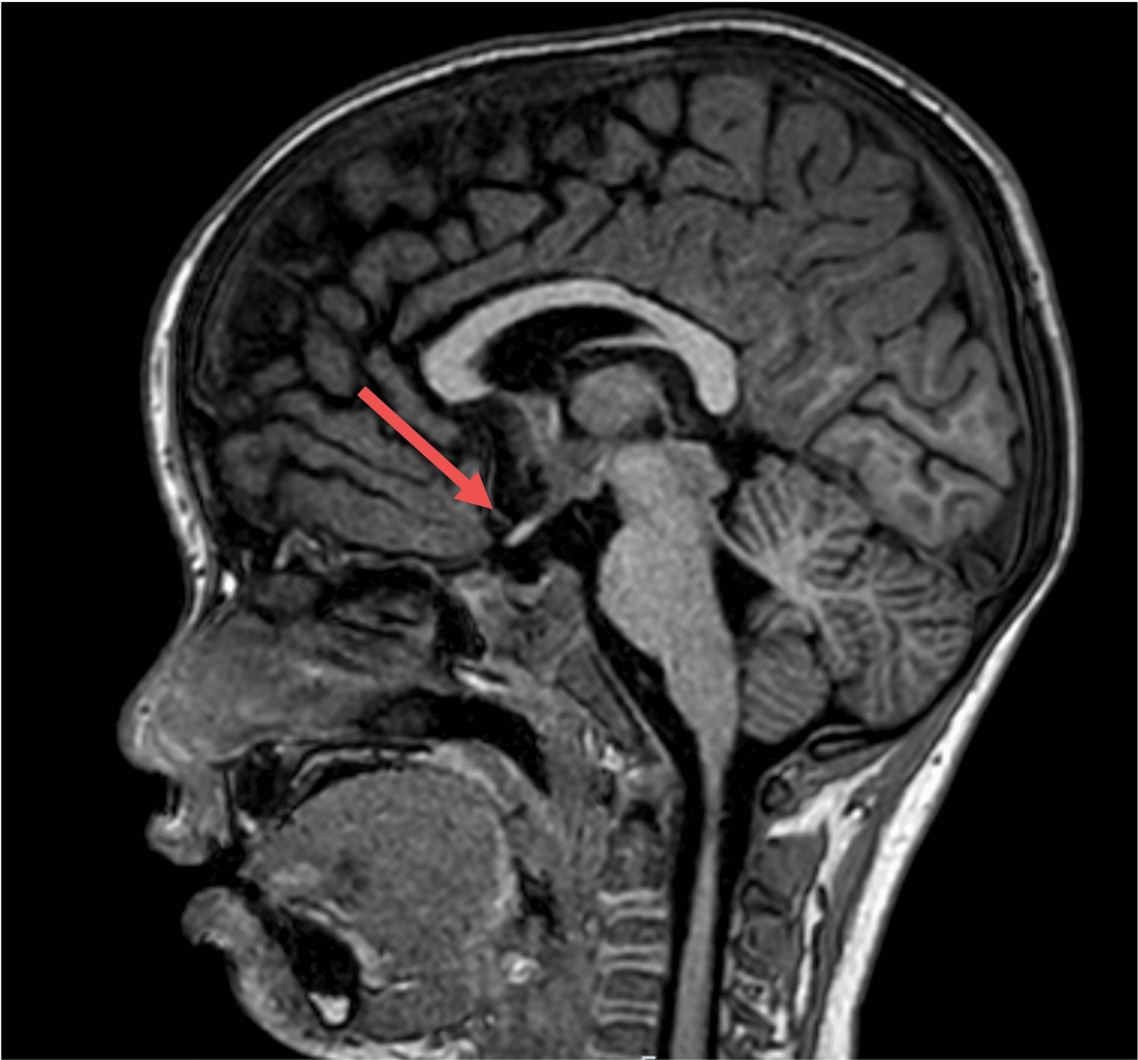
Post-therapeutic magnetic resonance imaging of the brain Magnetic resonance imaging of the head showed a reduction in the empty sella.
